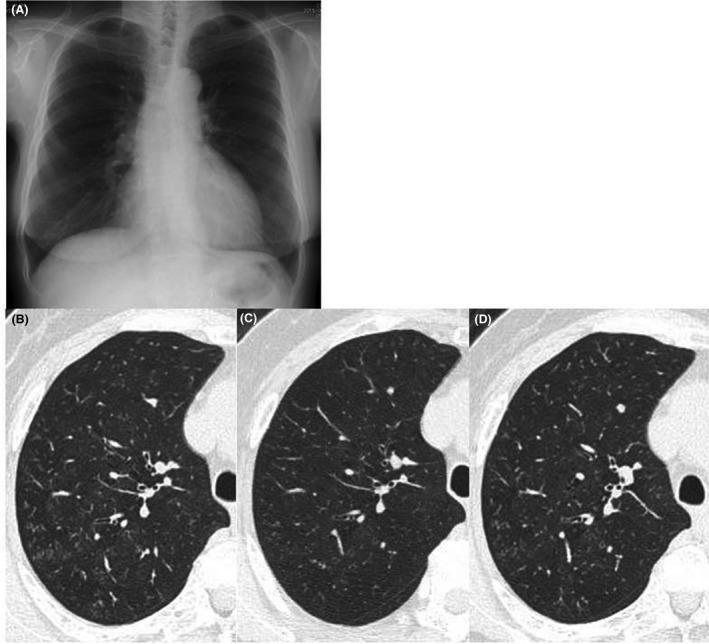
Chest imaging. Chest X‐ray on initial presentation showed no abnormal findings (A), but chest computed tomography showed centrilobular nodules and ground‐glass opacities (B). After the patient stopped working at the koji brewery, the abnormal shadows improved on computed tomography (C); however, they relapsed after she returned to the brewery (D).
